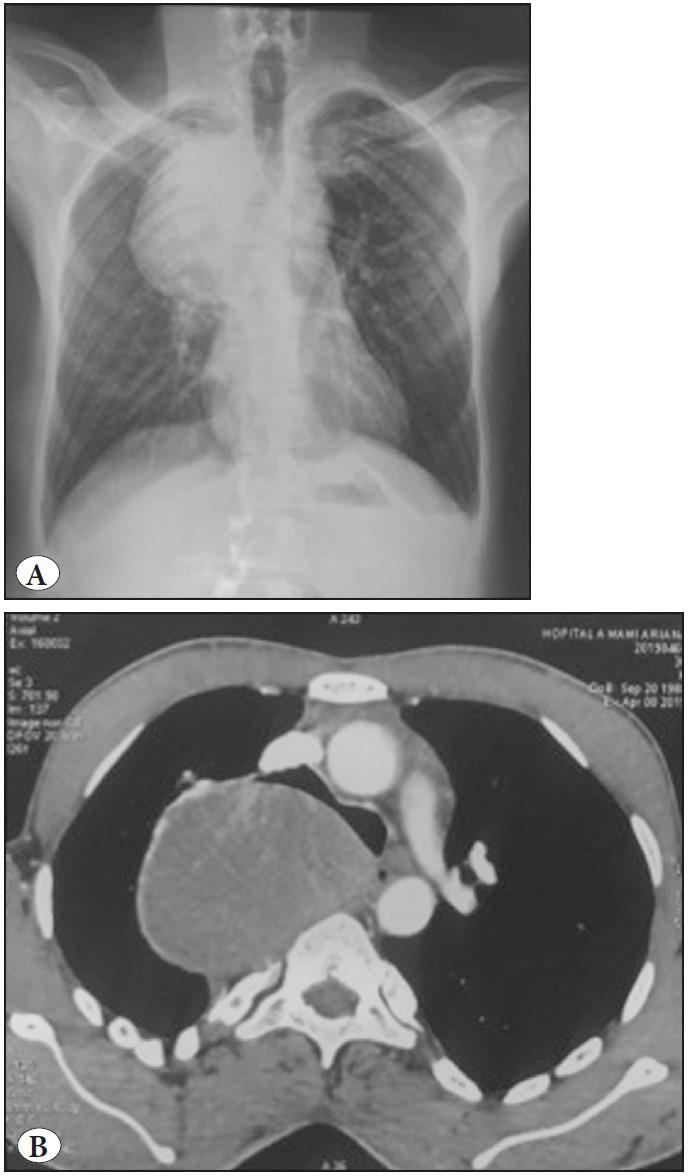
A) Chest-X-ray showing a 10 cm latero-tracheal shadow. B) CT-scan revealing a posterior and mediastinal mass in contact with D2 and D4 measuring 82x68 mm without costal invasion or lymph node metastases.
